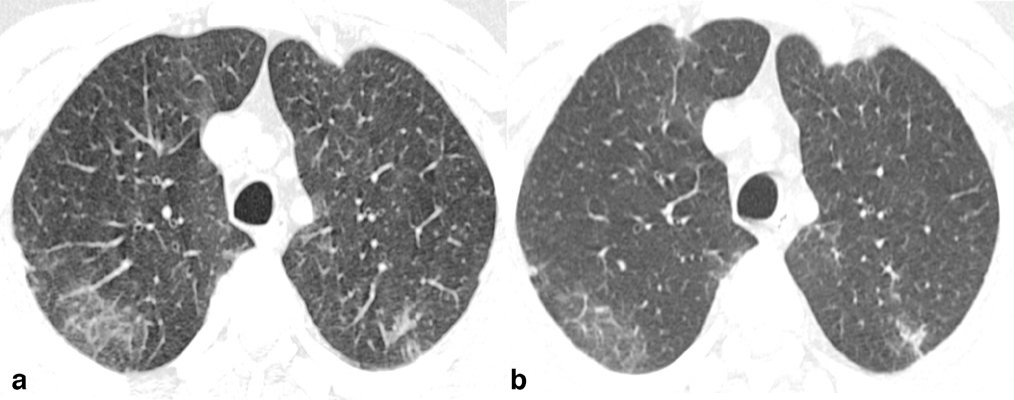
Axialchest CT images in lung window settings of a 51-year-old COVID-19 asymptomaticpatient, obtained 6 days apart, reveal comparable density and similar sizedGGOs with reticulations in subpleural lung in posterior segment of bilateralupper lobes.
